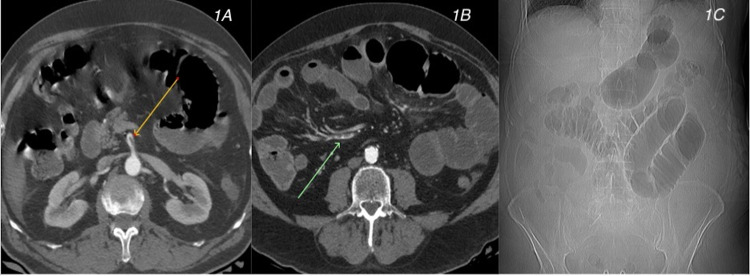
Case 1. CT angiography shows intraluminal thrombus within the celiac trunk (yellow arrow in 1A) and ileocolic artery (green arrow in 1B). CT (scout view) (1C) shows focal small bowel ileus compatible with ischemia. CT, computed tomography
